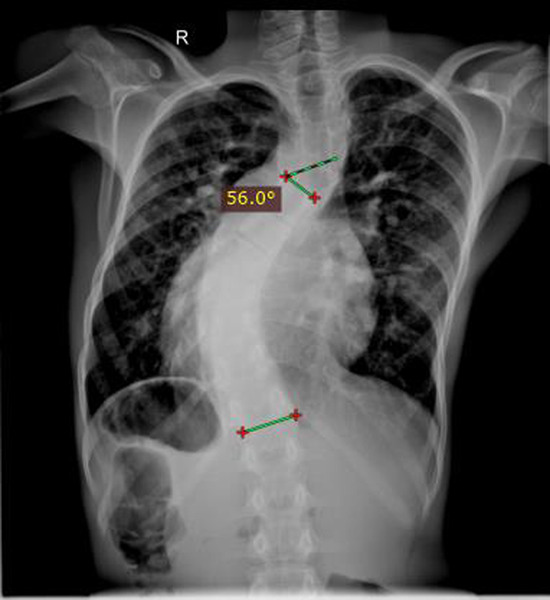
A plain chest radiograph of a 13-year-old boy with Cobb angle measurement of 56^0^
